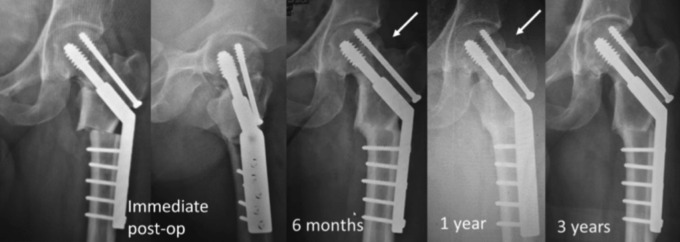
Left hip radiographs after subtrochanteric sliding osteotomy and DHS fixation. Union achieved over osteotomy site (cortical bridging) at six months while femoral neck non-union site (arrow) showed progressive union at three years (fracture line disappearance).
